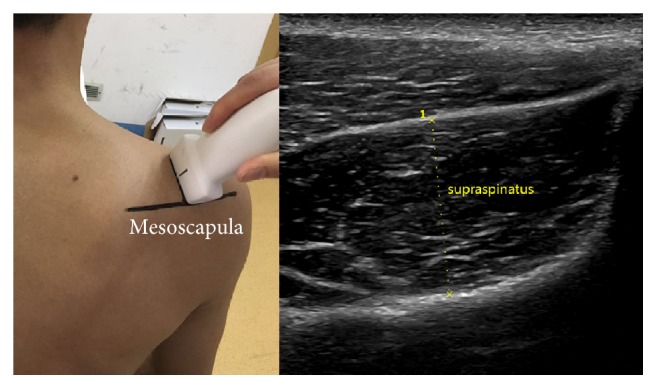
Anatomical structures: mesoscapula; the position and orientation of transducer; and the ultrasound image: SST (supraspinatus thickness).
